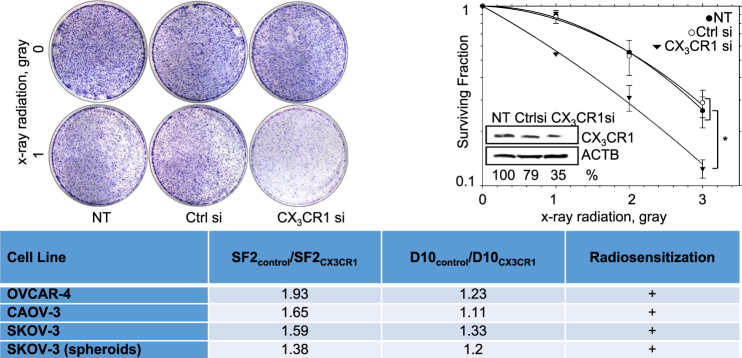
Downregulation of CX_3_CR1 synergizes with x-ray radiation to reduce clone formation of HGSOC cell lines. OVCAR-4 was transiently transfected with either CX_3_CR1-specific (designated “CX_3_CR1si”) or control (designated “Ctrl si”) si RNAs or vehicle (designated “NT”) and subjected to 0, 1, 2, or 3 gray x-ray radiation on the third day following transfection. Images of cells subjected to 0 or 1 gray and subsequently used in clonogenic assay are shown. CX_3_CR1 expression was determined by western blot; ACTB was used as loading control, and normalized expression of CX_3_CR1 was calculated using digital densitometry. Survival curves are the average of five independent experiments. p* < 0.05, two-way ANOVA test. Radiosensitization shown in the table was determined for OVCAR-4, Caov-3, and SKOV-3 (cultured as monolayer and spheroid cultures) if both SF2_control_/SF2_CX3CR1_ and D10_control_/D10_CX3CR1_ were >1.1
